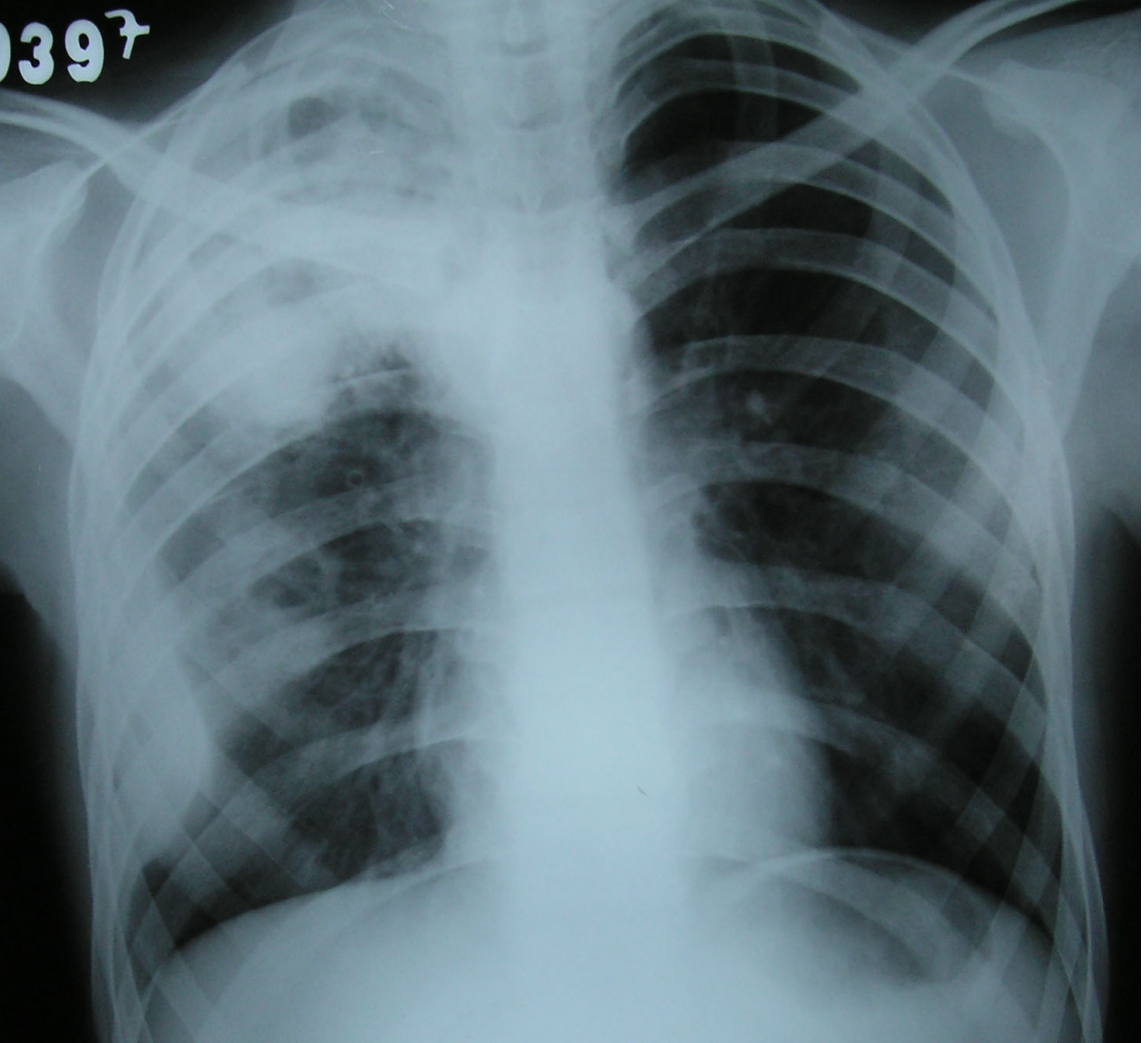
A 5 × 6 cm circular lesion located in the apex of the right lung at X-ray.
